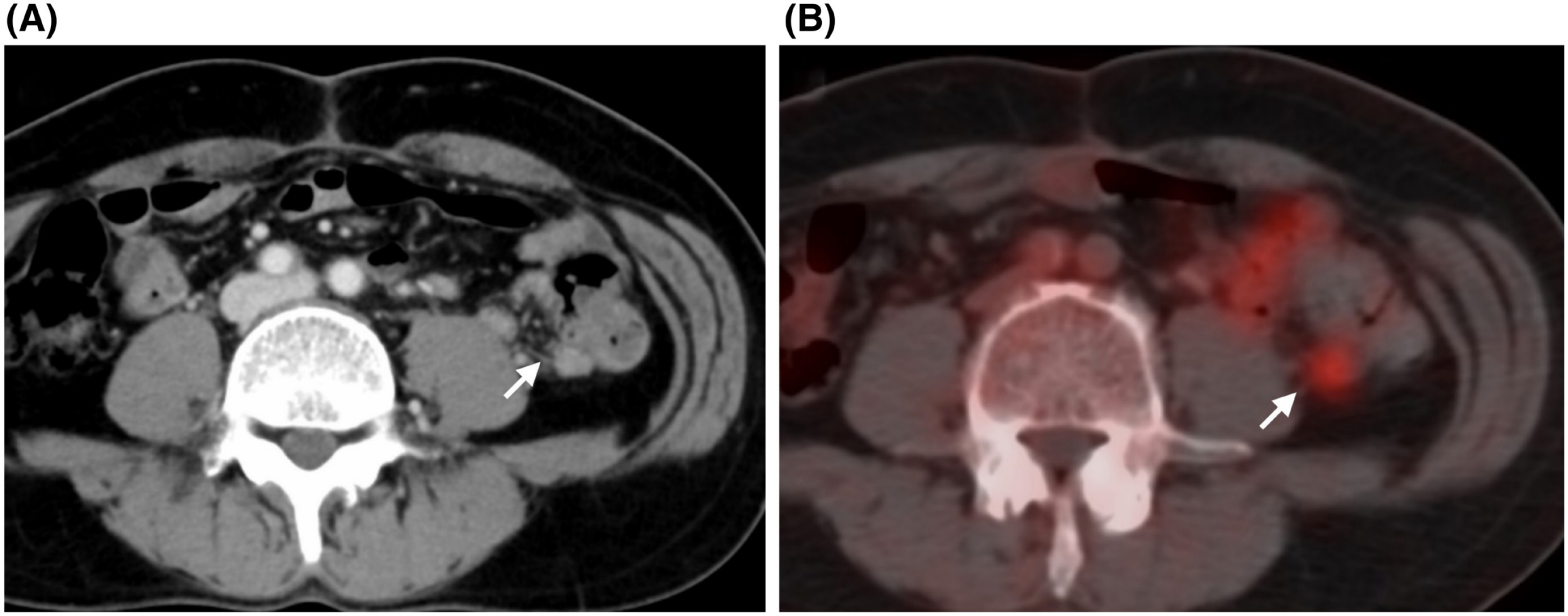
CECT scan and 18F‐FDG‐PET/CT findings in Case2. CECT scan indicates a tumor formation (10 × 11 mm) at the dorsal side of the anastomotic site of descending colon. (A, white arrow), corresponding to the SUVmax 3.4 tumor in the 18F‐FDG‐PET/CT scan (B, white arrow)
